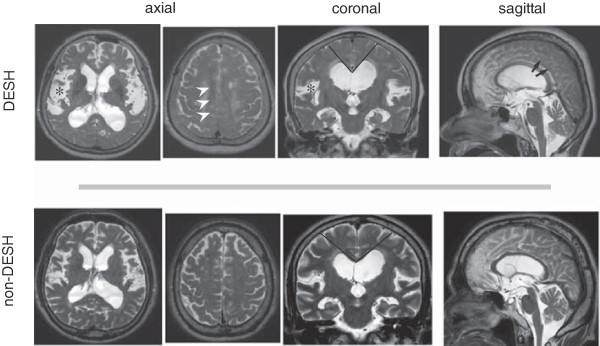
Representative magnetic resonance images of disproportionately enlarged subarachnoid-space hydrocephalus (DESH, upper row) and non-DESH ventriculomegaly (bottom row). Asterisks mark dilatation of the Sylvian fissure. Arrow heads, tight high convexity; Bars, callosal angles; Arrows, tight medial parietal sulci.
